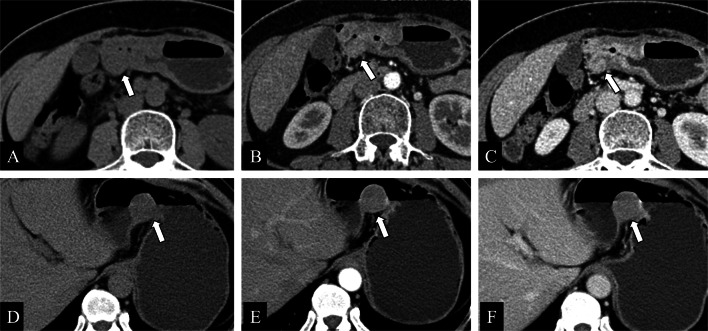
a–c Heterotopic pancreas in a 61-year-old female. Axial contrast-enhanced CT images obtained during the plain (a) /arterial (b) /venous (c) phases showed a heterogeneous enhanced flat mass (white arrows) in the gastric antrum. The mass had an endoluminal growth pattern, which is more common with heterotopic pancreatic lesion in the stomach. d–f Gastrointestinal stromal tumor in a 41-year-old male. Axial contrast-enhanced CT images obtained during the plain (d) /arterial (e) /venous (f) phases also showed a homogeneous enhancing round mass (white arrows) in the gastric antrum with the same endoluminal growth pattern
